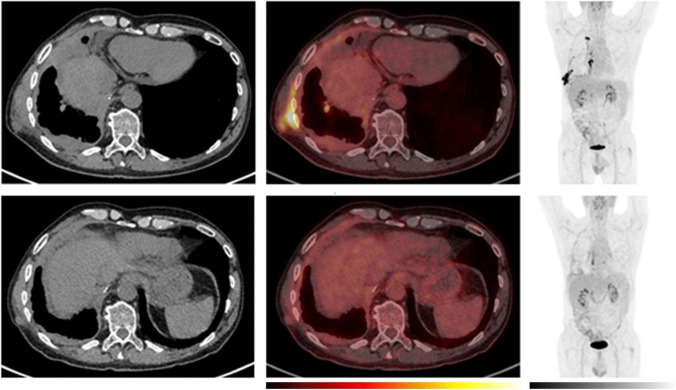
Example of a patient with a decline in MTV and [^18^F]fluorodeoxyglucose (FDG) uptake as early as the week-5 PET scan which was followed by a long-term objective tumour response. Comparative imaging from baseline (top row) and week-5 (bottom row). To the left, axial computer tomography (CT) images, in the middle, axial positron emission tomography (PET)/CT images and to the right the maximum intensity projection (MIP). The PET images are observed within a 0-10 SUV window. The patient was classified as having stable disease at the first CT response assessment, before developing partial response at the week-12 CT scan according to the modified Response Criteria in Solid Tumours (mRECIST) and immune RECIST. While the patient had stable disease at the week-5 CT scan, PET/CT already showed a reduction in metabolic tumour volume (MTV) and a decline in [^18^F]FDG uptake in the tumour. The treatment was discontinued after 7 months due to intolerable toxicity. The patient is still under follow-up 20 months after the start of the treatment
